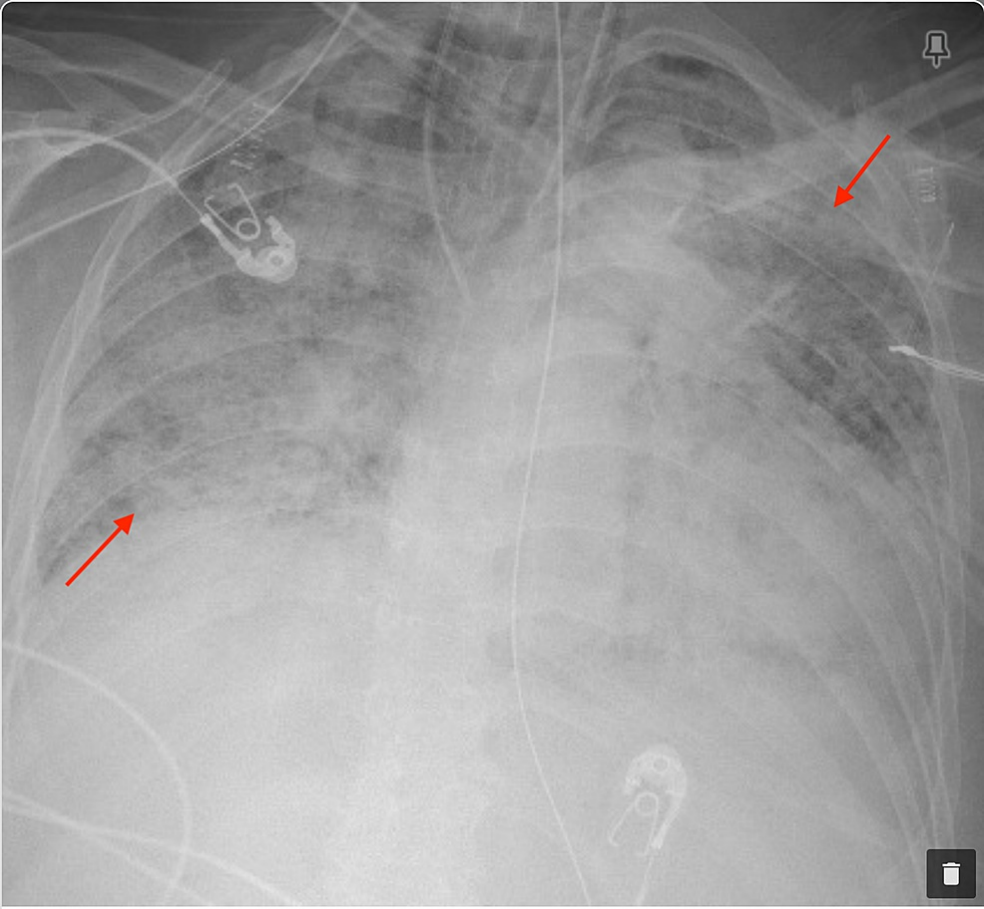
Frontal chest X-ray during hospitalization before starting plasmapheresis showed diffuse alveolar infiltrates bilaterally progressed from prior study compatible with worsening of alveolar hemorrhage or pulmonary edema
